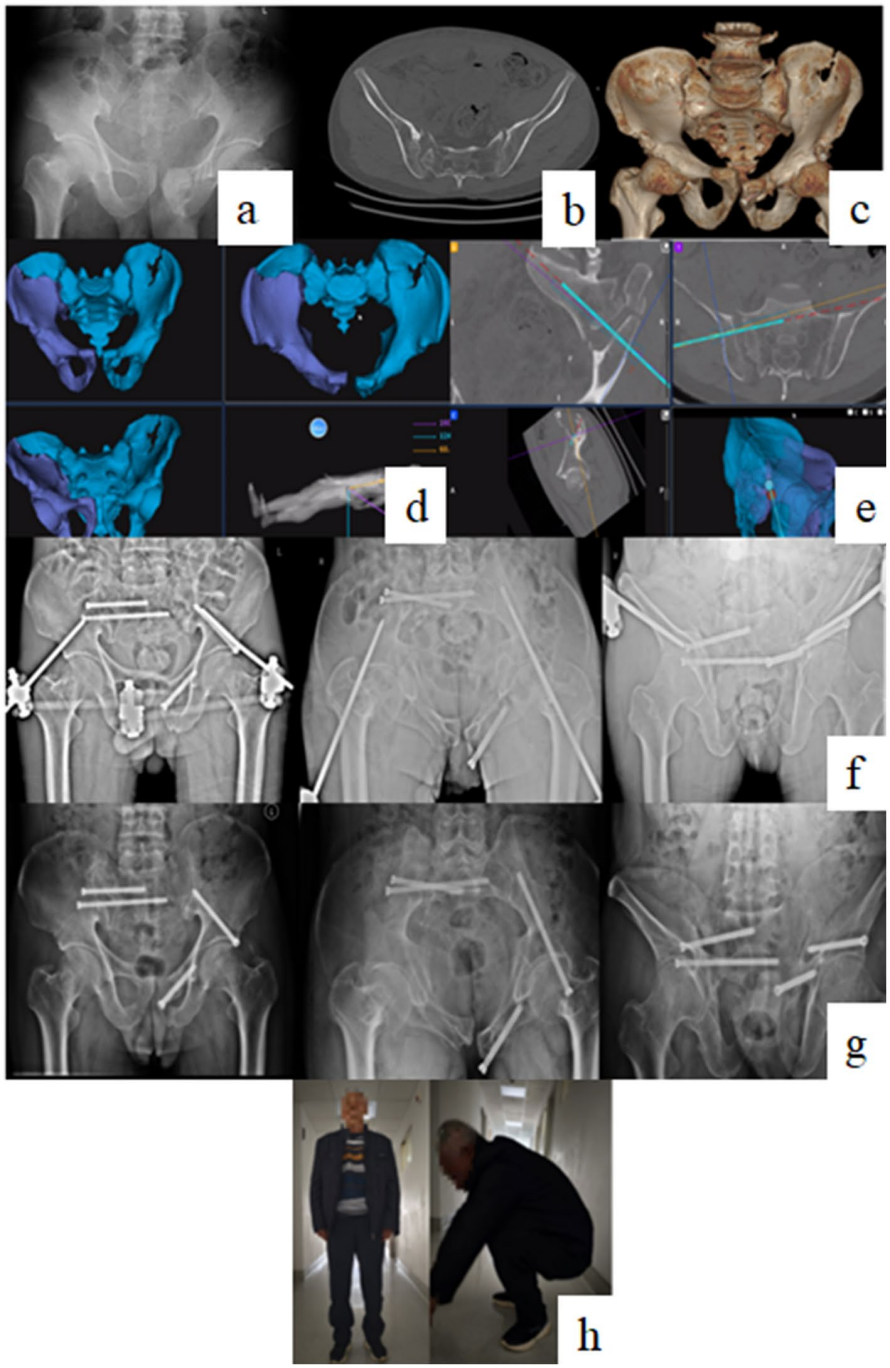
Representative clinical case of a Tile C pelvic fracture. (a–c) Preoperative radiographs, CT, and 3D reconstruction images; (d) Intraoperative reduction under navigational monitoring; (e) Guidewire placement for sacroiliac screw under navigation following reduction; (f,g) 1-month and 3-month postoperative follow-up radiographs; and (h) Lower limb functional status postoperatively.
